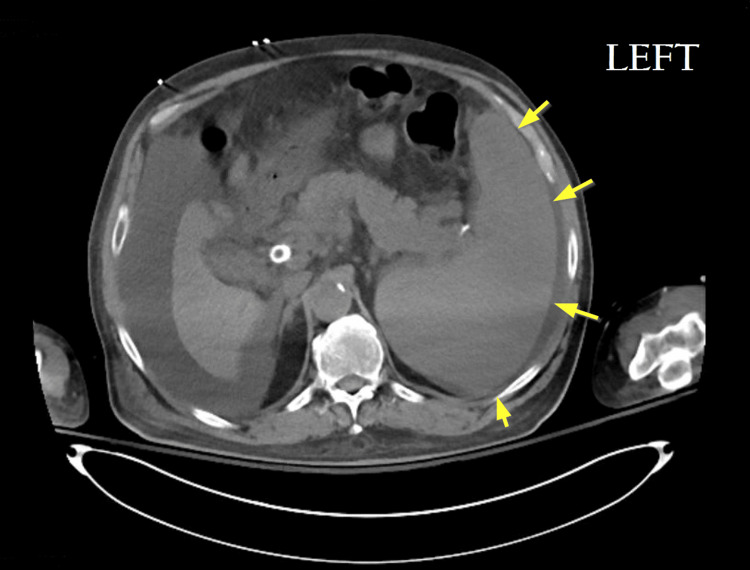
Axial CT image of the spleen before partial splenic embolization. Pre-embolization CT demonstrates splenomegaly with estimated splenic dimensions of 14 × 19 × 19 cm and a calculated total volume of approximately 3,032 mL (yellow arrows).
